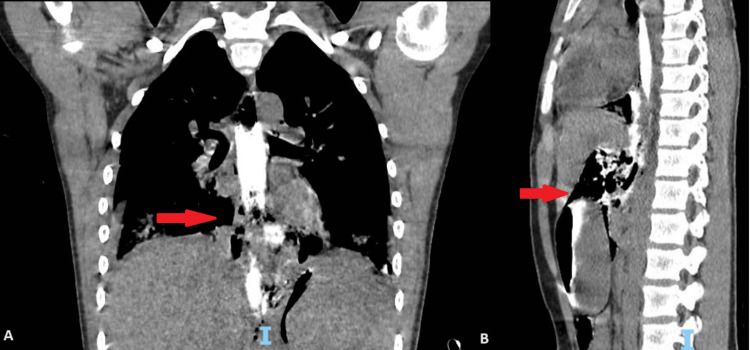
CT with oral contrast in coronal (A) and sagittal (B) views showing a contained leak in the periesophageal area.
